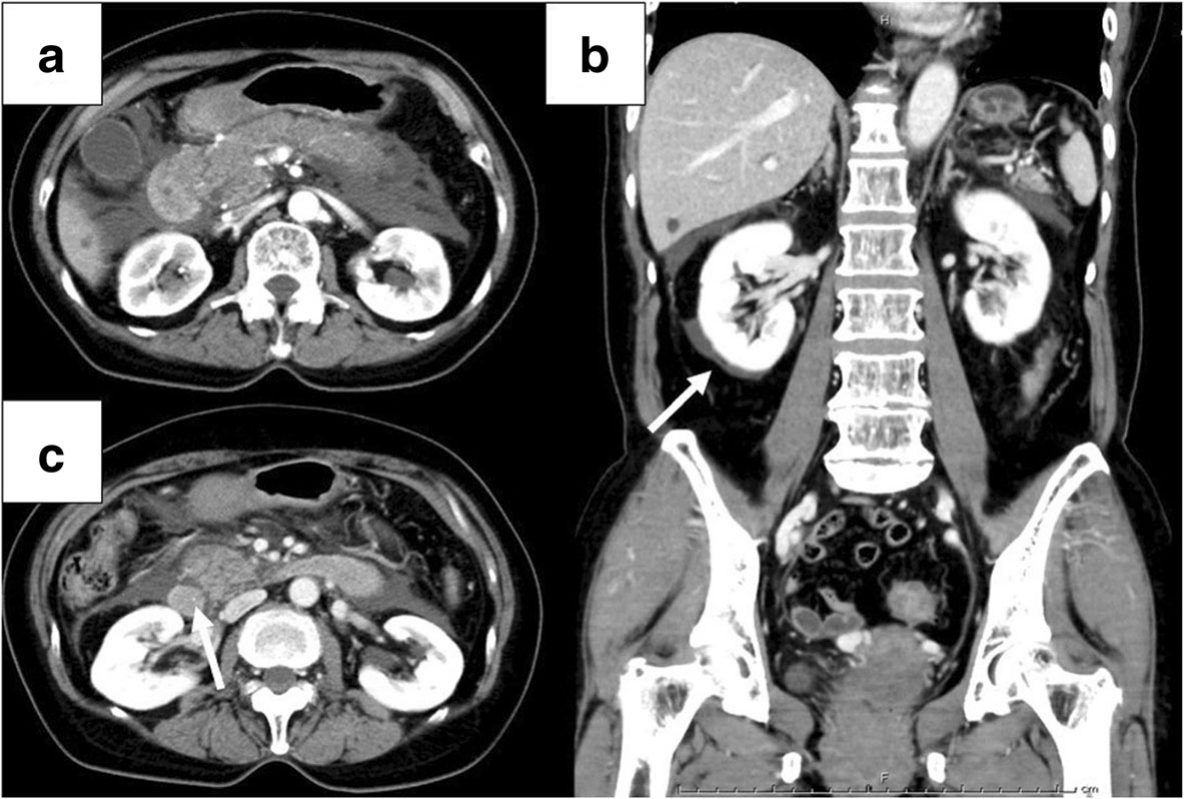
a Abdominal contrast-enhanced CT showed pancreas enlargement, especially in the head of the pancreas. The pancreas showed homogenous enhancement. b Abdominal contrast-enhanced CT revealed fluid collection around the pancreas spread beyond lower kidney edge (white arrow). c There was a common bile duct stone (white arrow)
